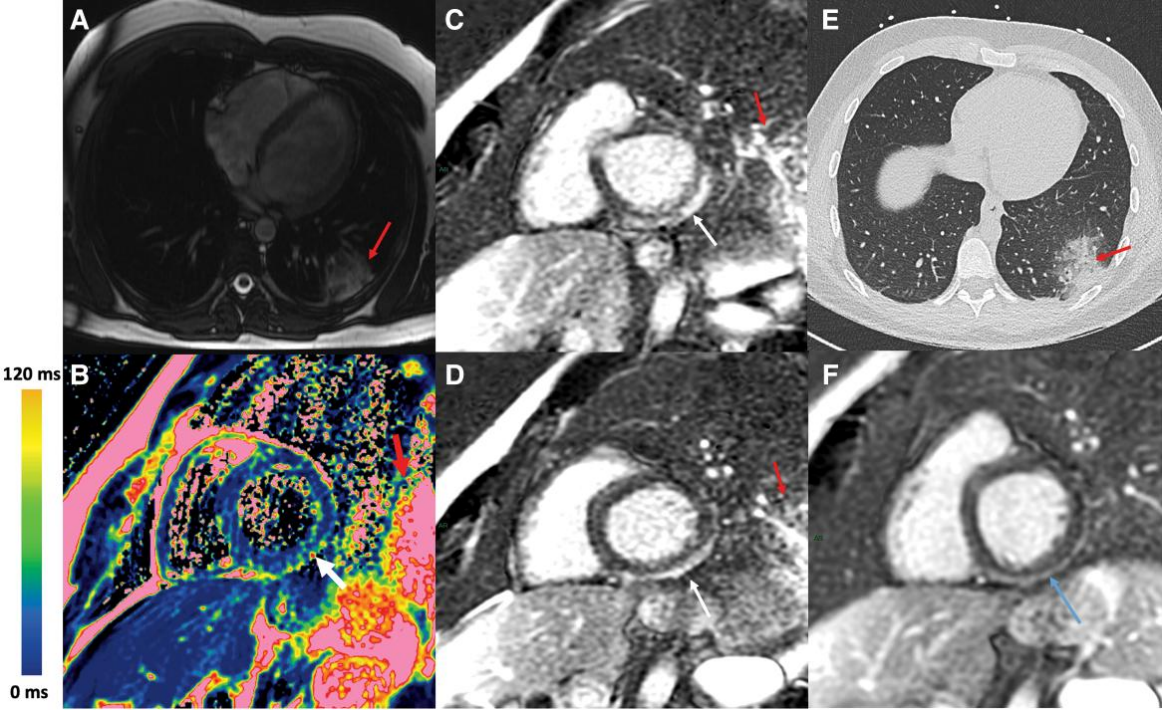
Myocarditis with pneumonia. (*A*) Trans-axial Fiesta localizer sequence (CMR). Presence of a left lower lobe consolidation (red arrow). (*B*) T2 mapping sequence (CMR). Presence of a T2 elevation in the subepicardial region of the inferolateral wall (white arrow). (*C* and *D*) LGE sequence. Presence of a subepicardial enhancement in the inferolateral region (white arrow). The left lower lobe consolidation can also be seen (red arrow). (*E*) CT scan showing left lower lobe consolidation. (*F*) Six-month CMR showing persistent LGE.
